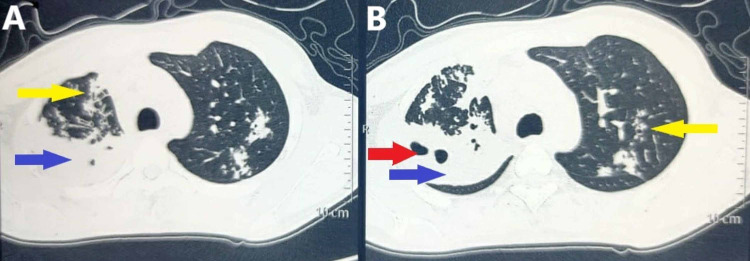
Axial sections (A and B) of HRCT scan of the chest showing consolidation (blue arrows) and cavity (red arrow) in the right upper lobe along with bilateral branching centrilobular nodules (yellow arrows). HRCT: High-resolution computed tomography
